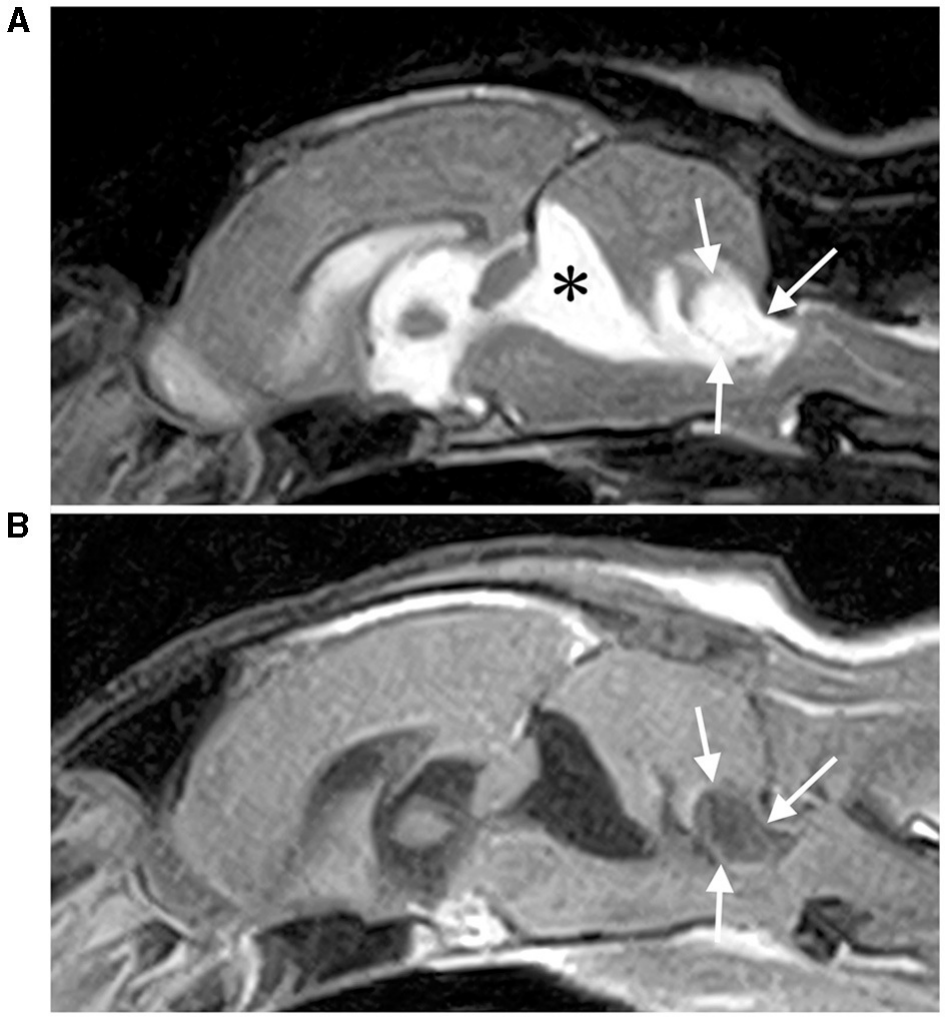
T2-weighted (A) and contrast-enhanced T1-weighted (B) sagittal MRI of the brain. An extra-axial cystic mass (white arrows) is observed caudal to the fourth ventricle and is compressing the fourth ventricle and medulla severely. A dilation of a second cystic lesion was also observed rostral to the cerebellum (*), which was considered an arachnoid diverticulum.
